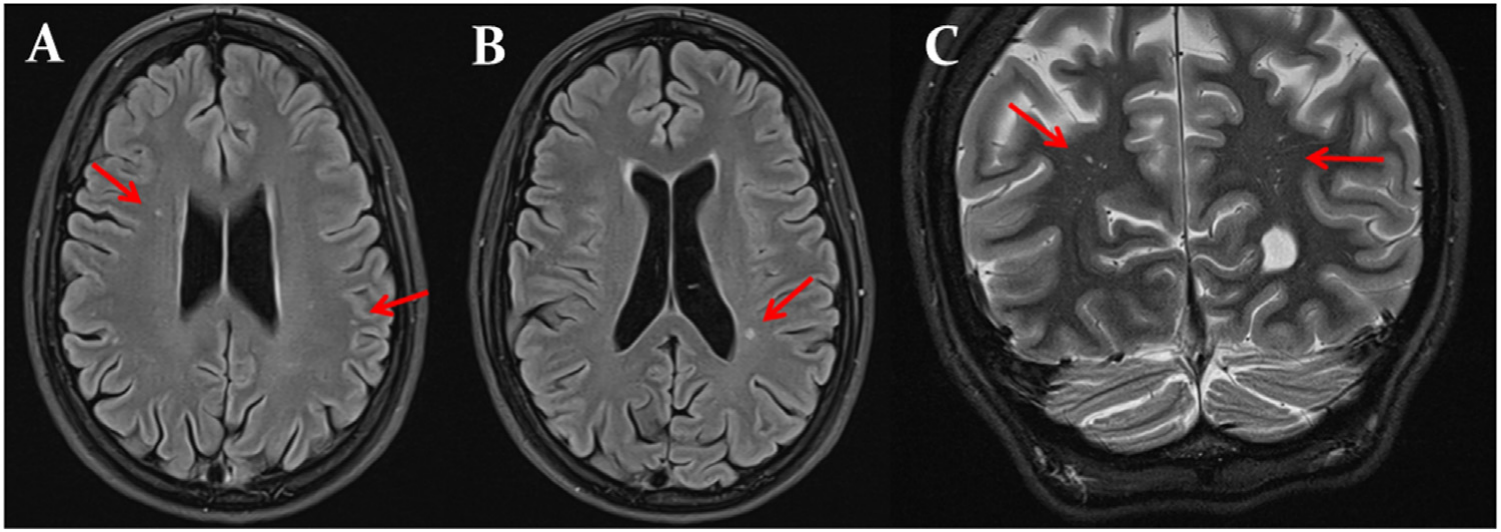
Multimodal cerebral MRI at the age of 32 shows mild global atrophy and multiple T2-yperintense spots within the white matter bilaterally (panels A, B, and C).
